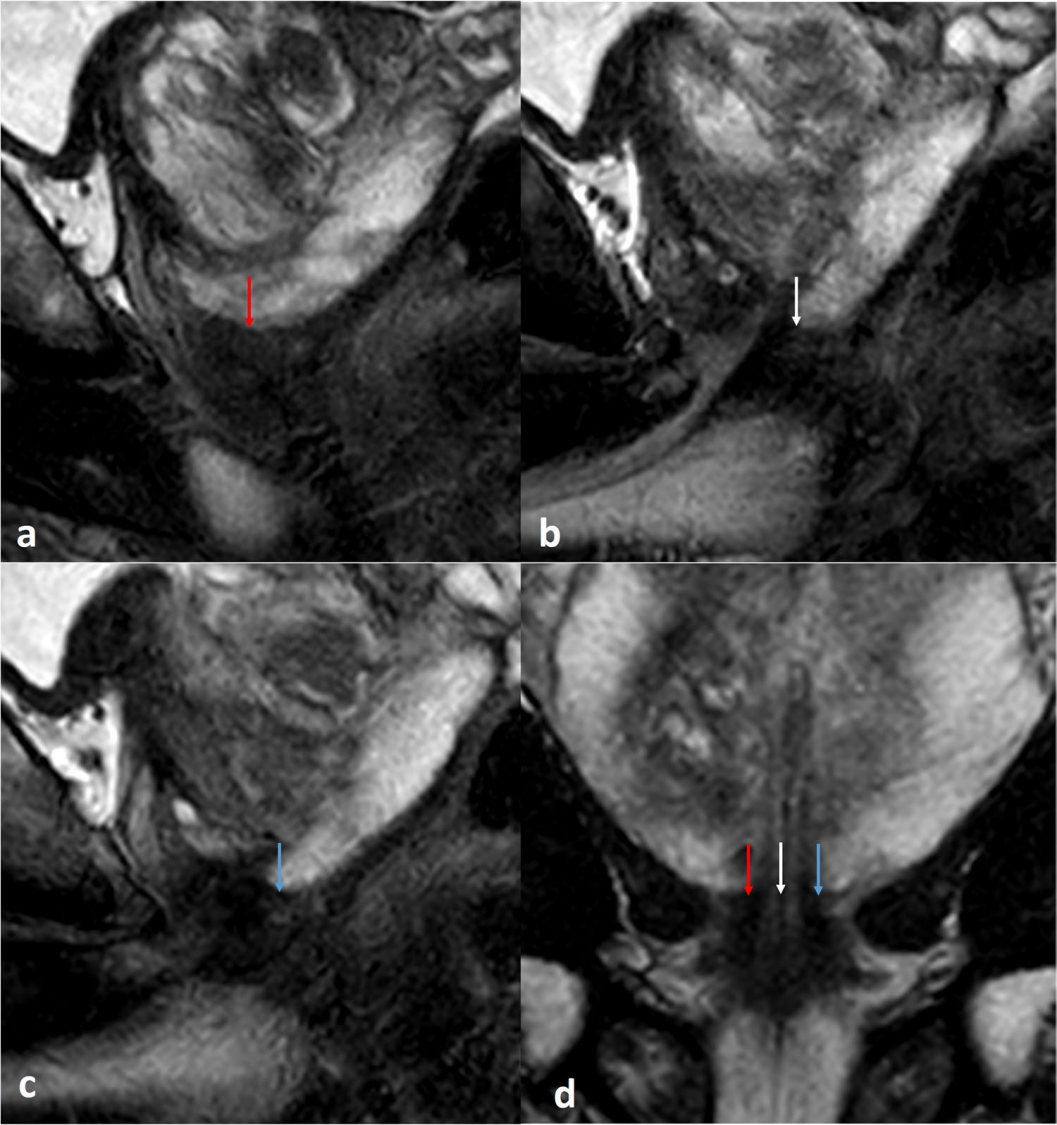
Challenges in membranous urethral length (MUL) measurement — the upper border, intersecting with the peripheral zone (1). Identifying the lower border of the peripheral zone may be challenging. Scrolling through the sagittal T2w images may help in determining the upper border of the MU, by better identifying the lower border of the peripheral zone. At the upper border of the membranous urethra, the retroprostatic part of the rectovesical space surrounding the peripheral zone in the prostate apex could be difficult to distinguish from the peripheral zone. These structures have similar signal intensities to the peripheral zone on T2-weighted images, especially when peripheral zone is less hyperintense on T2w images due to inflammation, fibrosis, blood products, or cancer. Scrolling 1 or 2 slices right (a; red arrow) and left (c; blue arrow) from the midsagittal view (b; white arrow) may improve the determination of the peripheral zone, and subsequently the upper border of the MU. On midsagittal images of the prostate, the dark tissue in the membranous urethra lumen may extend intraprostatic. This intraprostatic tissue will most likely be resected and should therefore be excluded from measurement. d Coronal T2 image shows the sagittal orientation of the lower borders of the peripheral zone on right parasagittal image (red arrow), midsagittal image (white arrow), and left parasagittal image (blue arrow)
